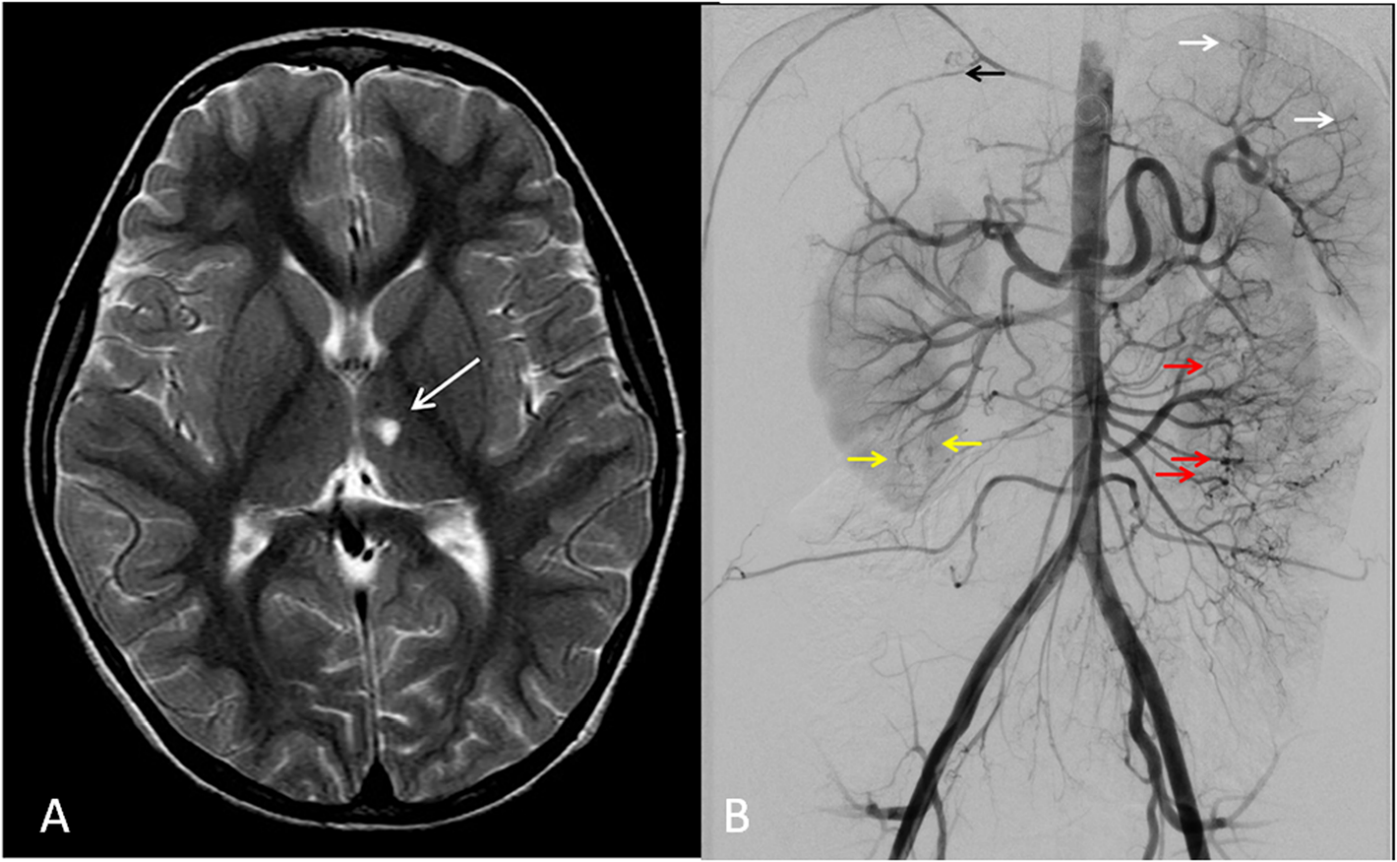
A 12-year-old male with deficiency of adenosine deaminase type 2 (DADA2) and long history of recurrent fever, left thalamic stroke at 5 years of age, livedo reticularis, and erythema nodosum: a Axial T2-weighted image demonstrates high signal left thalamic lesion with mild negative mass effect (arrow), no surrounding edema and no diffusion restriction (not shown), indicative of old infarct. b Aortic angiogram through a right femoral puncture access: pruning, distal obliteration and irregularity of the intra-hepatic arteries (black arrow). There are multiple microaneurysms in the distal branches of the splenic artery (white arrows) and intra renal arteries of the right kidney (yellow arrows). There is hypervascularity of the jejunum with multiple irregularities and micro-aneurysms involving the jejunal branches (red arrows). Findings are in keeping with small vessel vasculitis such as polyarteritis nodosa (PAN). This patient tested positive for disease-causing gene mutation (CECR1)
